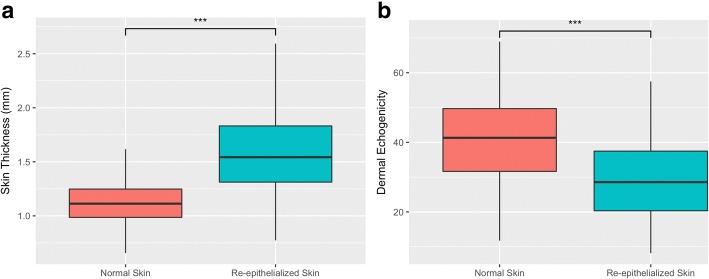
Differences in ultrasonographic findings between re-epithelialized skin after a partial-thickness burn and contralateral normal skin. a Difference of skin thickness. b Difference of dermal echogenicity. p*< 0.05, *p* < 0.01, p* < 0.001, ns = *p* > 0.05
